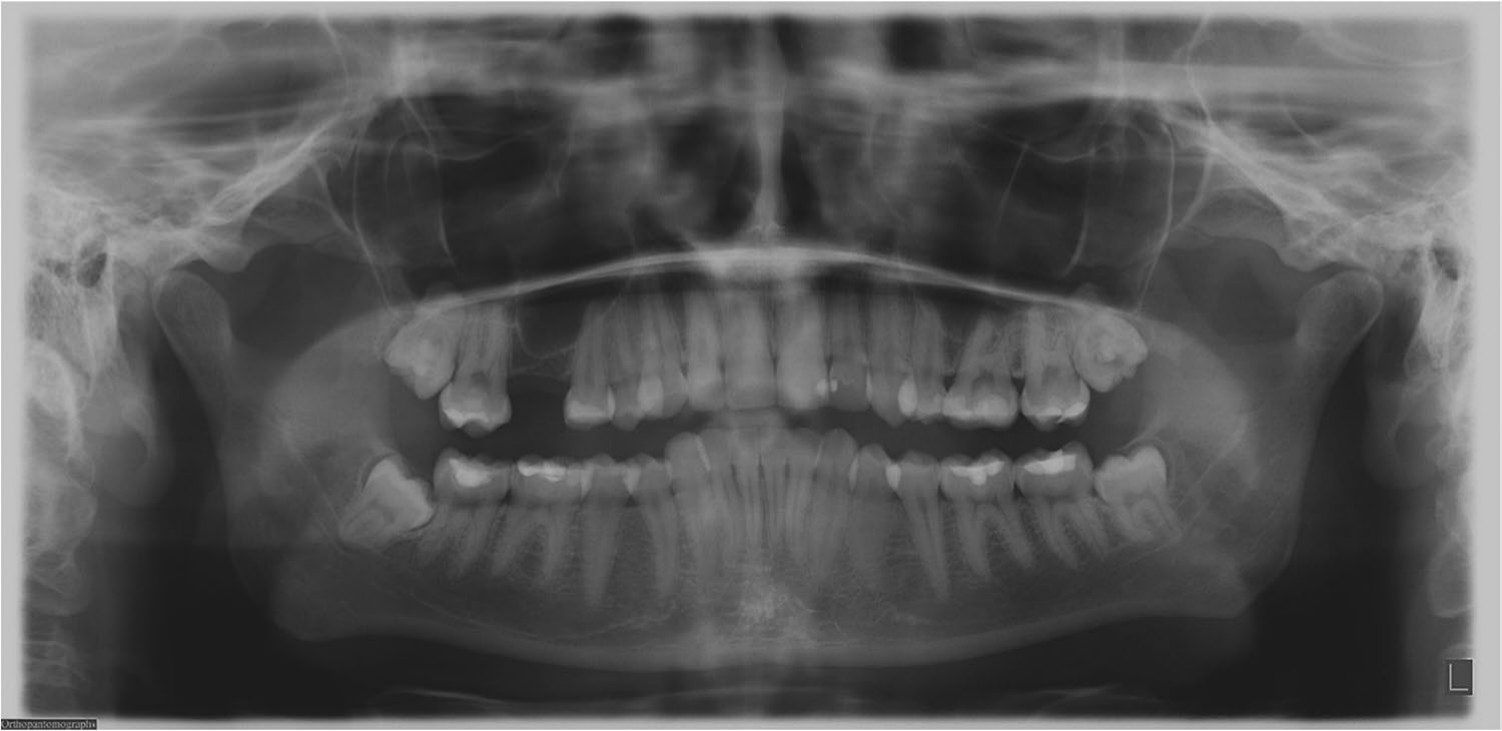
A preoperative panoramic radiograph of a 27-year-old female patient before the coronectomy of the right mandibular third molar
